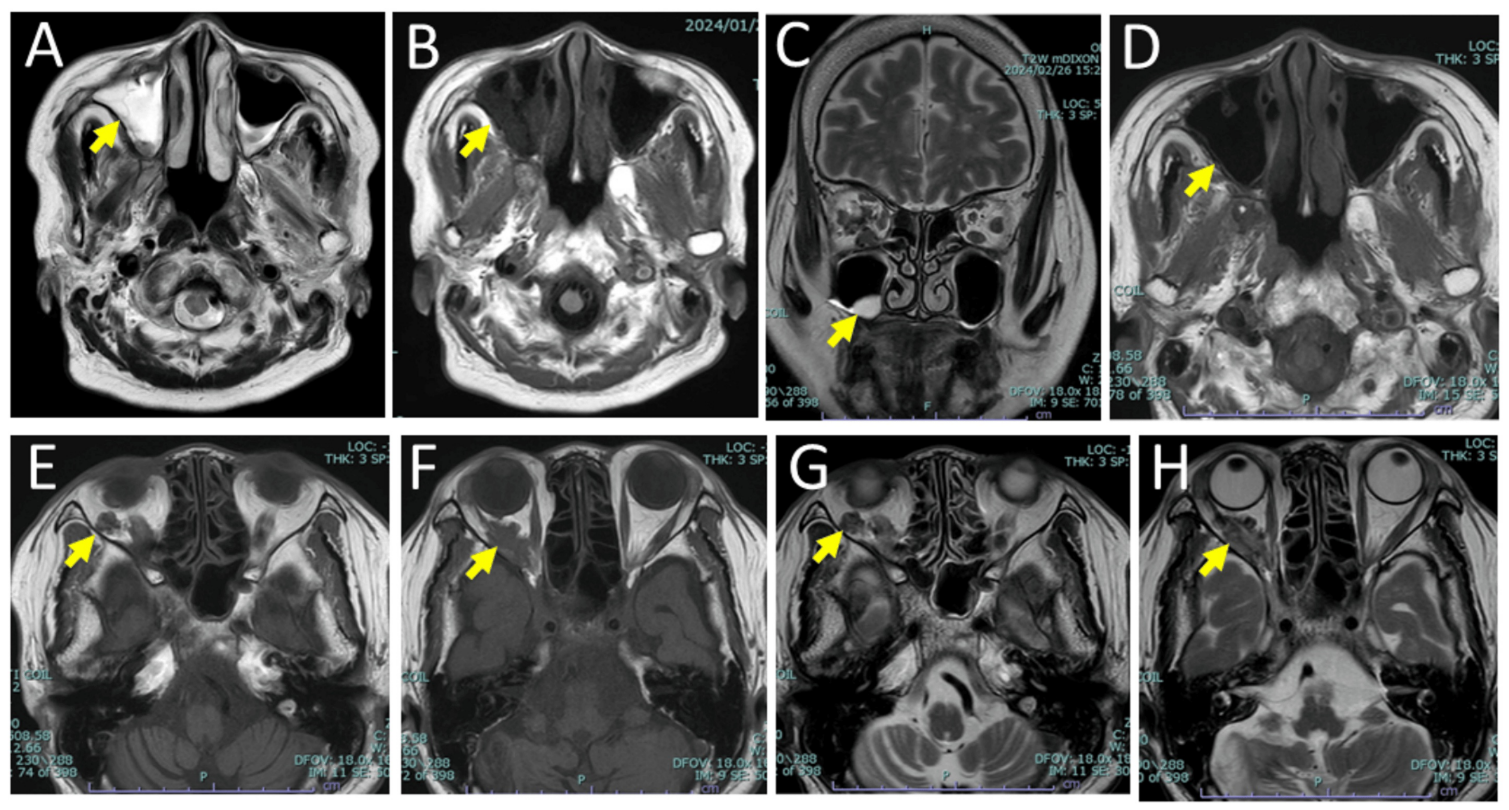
Magnetic resonance imaging on the next day at referral and one month later. Right maxillary sinusitis (arrows) in T2-weighted (A) and T1-weighted (B) axial images the next day at a referral hospital. Resolution of right maxillary sinusitis (arrows) in T2-weighted coronal (C) and T1-weighted axial (D) images in one month with penicillin G treatment. Note a small volume of residual fluid in the right maxillary sinus (arrow, C). Right orbital vascular malformation (arrows) in the pre-existing size and no proptosis after one month of antibacterial treatment in T1-weighted (E, F) and T2-weighted (G, H) axial images.
